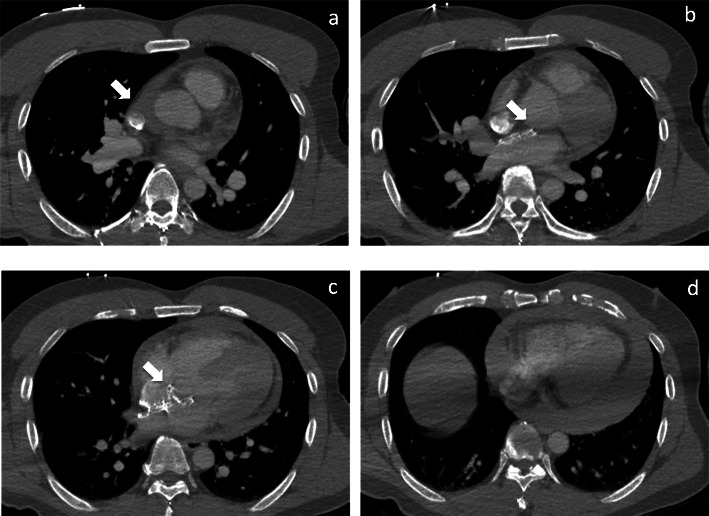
Computed tomography scan shows pericardial effusion with no evidence of aortic dissection. a Pericardial fluid accumulates near the transverse pericardial sinus, suggestive of erosion. b, c The edge of the Amplatzer septal occluder was in contact with the aortic wall from the anterior wall of the left atrium, adjacent to the aortic root. d The pericardial effusion was circumferential
